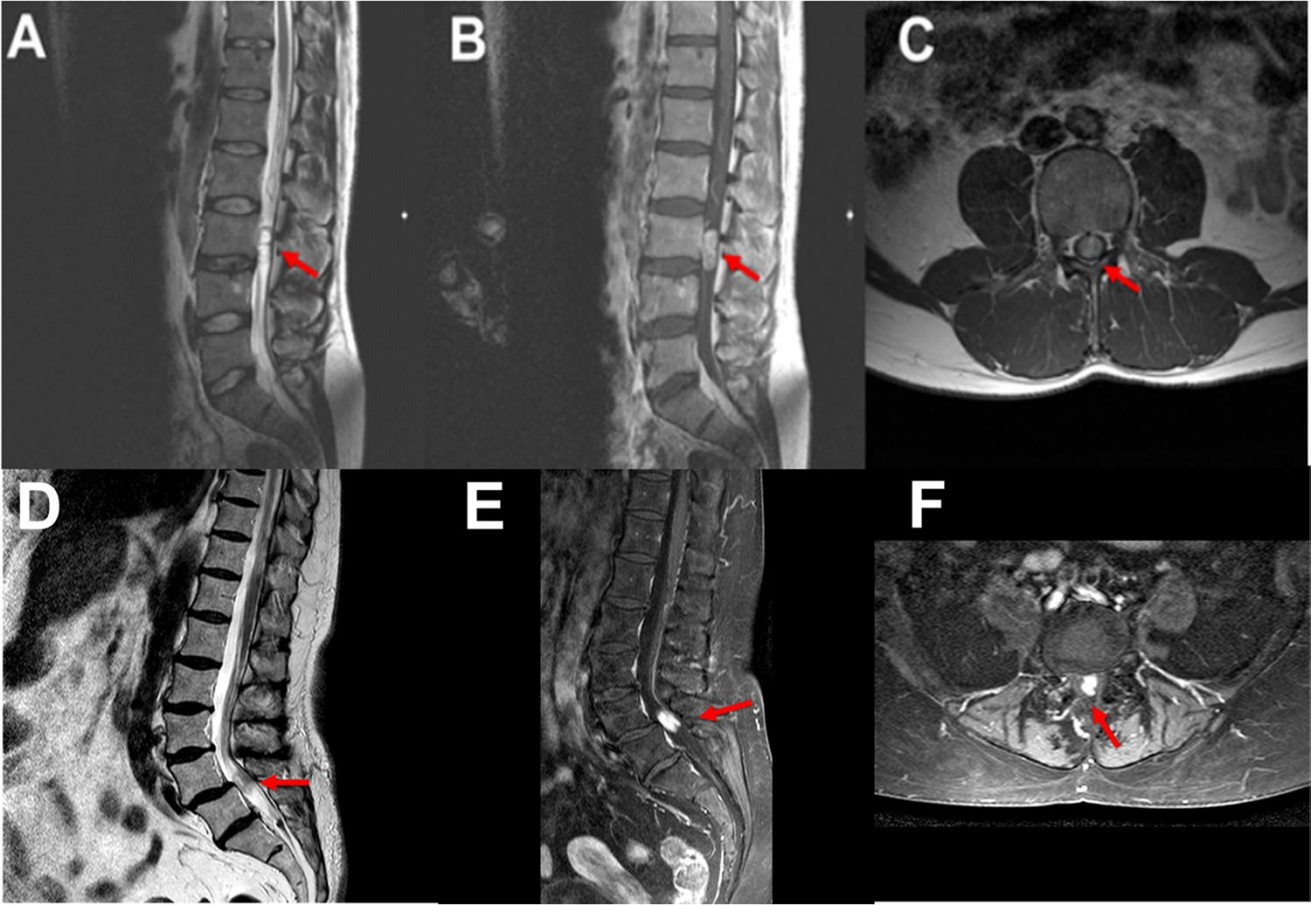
Representative MRI images of paragangliomas. A–C Patient number 5 (Table 1) images. A) Sagittal T2W image showing the intradural lesion (arrow). B) Sagittal post-contrast (Gadolinium) T1W image demonstrating homogenously enhancing lesion (arrow). C Axial post-contrast (Gadolinium) T1W image demonstrating an intradural homogenously enhancing lesion (arrow). D–F Patient number 9 (Table 1) images. D) Sagittal T2W image suspicious of a spinal lesion (arrow). E) Sagittal post-contrast (Gadolinium) T1W image demonstrating enhancing lesion (arrow). F) Axial post-contrast (Gadolinium) T1W image demonstrating an intradural enhancing lesion (arrow). Please note that in this patient (number 9), the post-contrast MRI was performed at a different date
